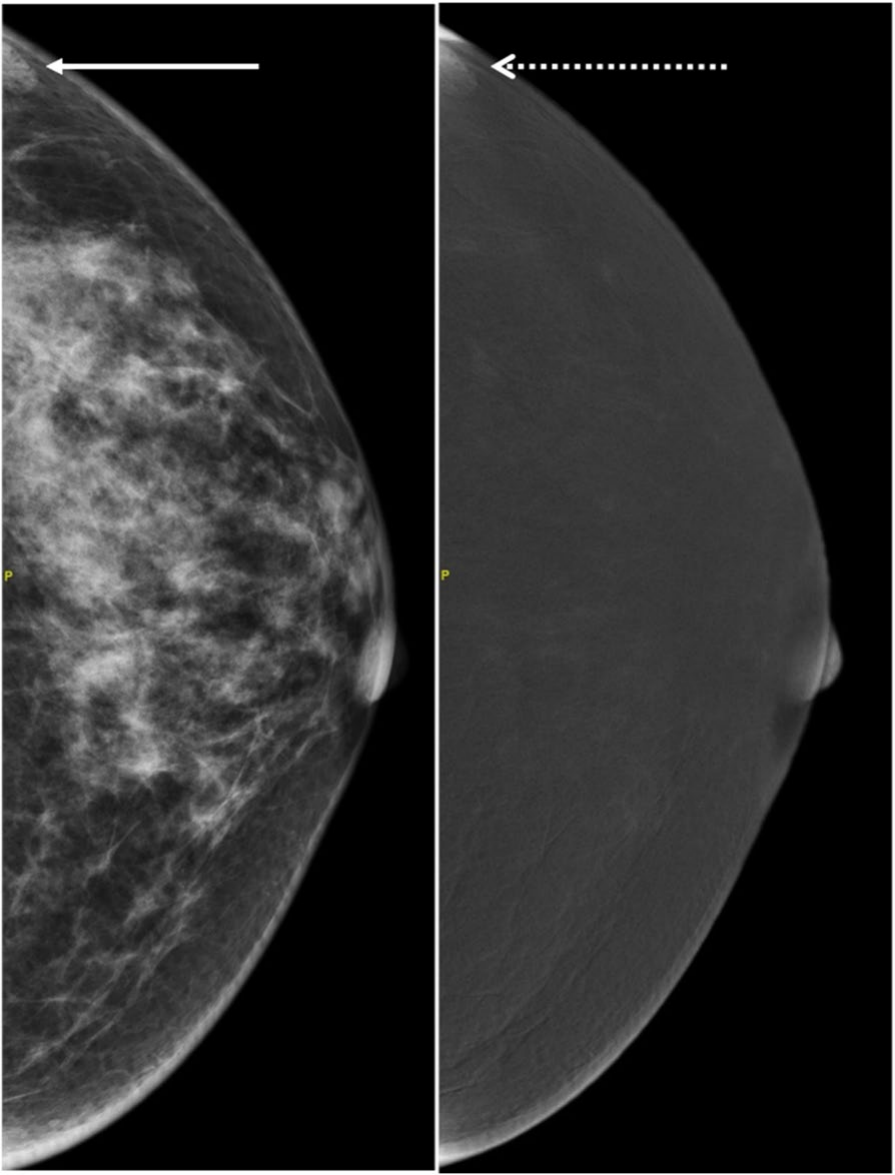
58-year-old woman presented for diagnostic work up for abnormal findings in both breasts. LE image shows an asymmetry (solid arrow) in the posterior far lateral left breast with corresponding enhancement on the RI (dash arrow). Upon physical exam at the time of ultrasound, this area of enhancement correlated to a skin lesion, seborrheic keratosis
